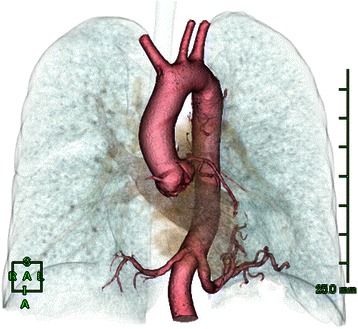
Three-dimensional CT revealed two aberrant arteries arising from the descending aorta and running into the lower lobes on each side, without a normal supply from the pulmonary artery
